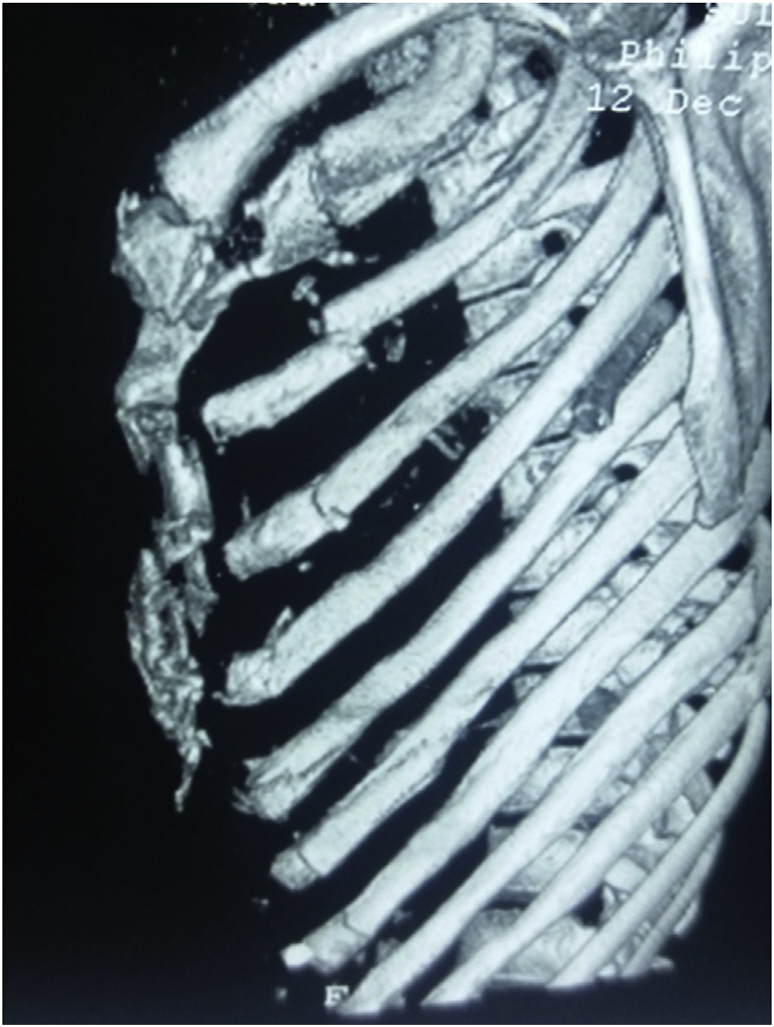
Three-D reconstructed computed tomography scan of a young female presented with blunt chest trauma demonstrating multiple rib fractures with sternal fracture.
